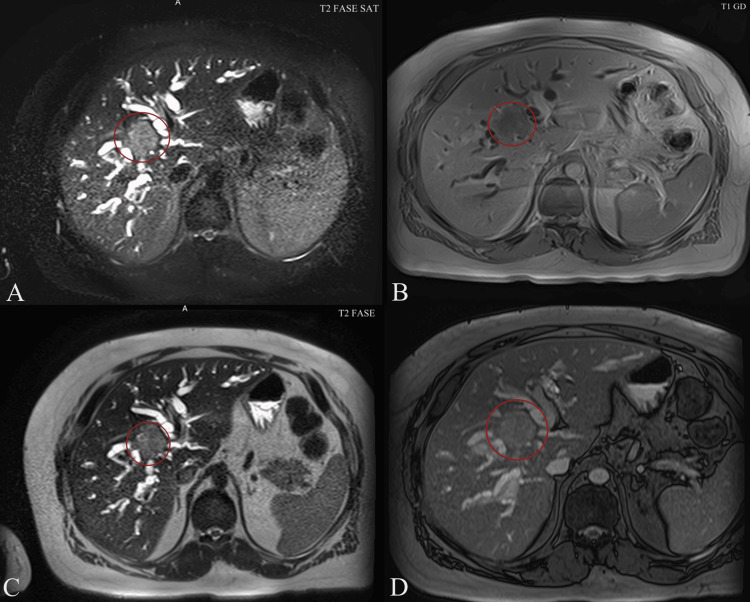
Magnetic resonance cholangiopancreatography (MRCP) and multiphasic MRI (axial planes) in a patient with Bismuth-Corlette type IIIa perihilar cholangiocarcinoma (Klatskin tumor). A) T2-weighted fat-suppressed sequence showing marked intrahepatic biliary dilatation with abrupt termination at the hepatic hilum (red circle). (B) T1-weighted gadolinium-enhanced arterial phase demonstrating mild heterogeneous enhancement of the hilar mass (red circle). (C) Standard T2-weighted sequence revealing bilateral intrahepatic duct dilatation and the “peripheral duct sign” (red circle). (D) T1-weighted gadolinium-enhanced delayed phase showing progressive delayed enhancement of the hilar tumor with adjacent capsular retraction (red circle), a finding highly suggestive of perihilar cholangiocarcinoma. Tumor classification is based on the Bismuth–Corlette classification system as originally described by Bismuth et al. (1992) [3].
